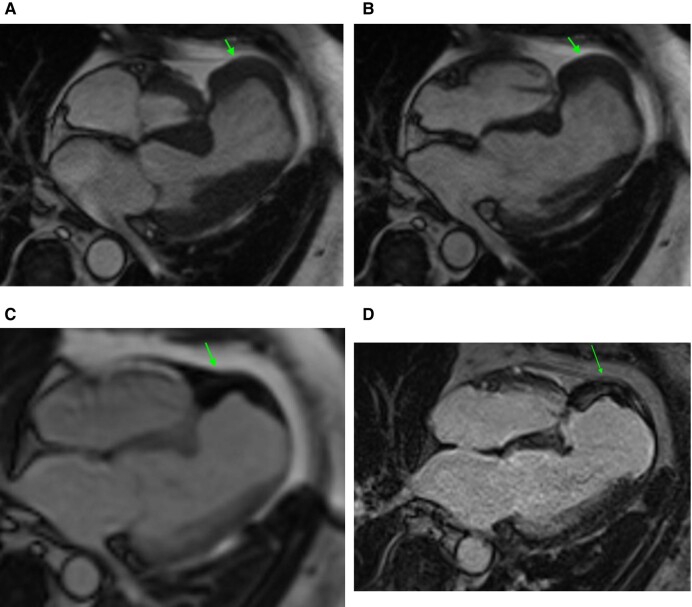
Cardiac magnetic resonance imaging. Pseudoaneurysm in each image is denoted by an arrow. Four-chamber view cine in systole (*A*) and diastole (*B*), with reduced function, measured LVEF of 33% and apical septal pseudoaneurysm. High TI imaging (*C*) with dark appearance of the dense material in the pseudoaneurysm, consistent with a thrombus. Delayed enhancement imaging (*D*) shows pseudoaneurysm containing thrombus at the apex of the left ventricle. The pseudoaneurysm measures 4.4 × 2.8 cm.
